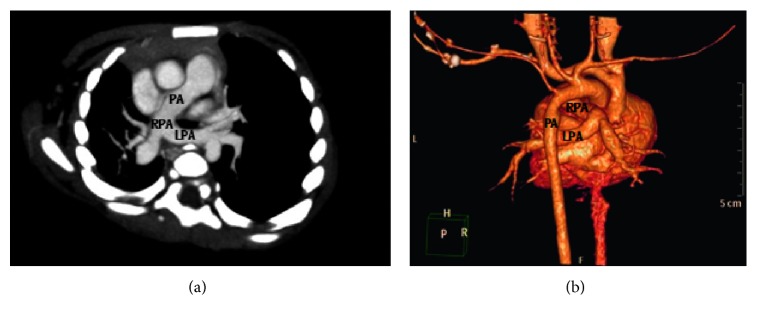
CT angiography (a) and 3D reconstruction (b) showing the anomalous origin of the left pulmonary artery (LPA) from the posterior aspect of the right pulmonary artery (RPA).
